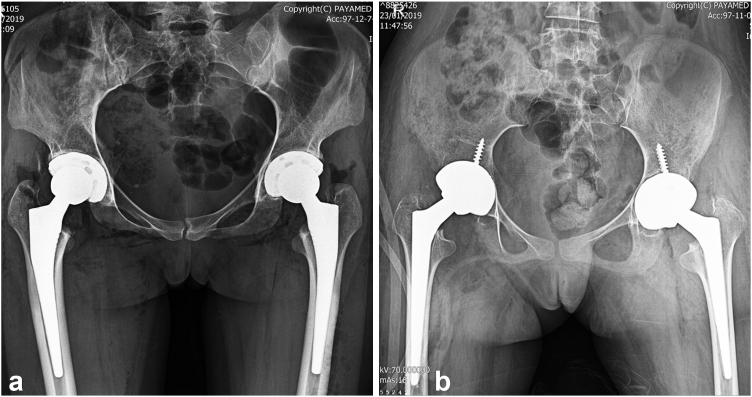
Postoperative anteroposterior pelvic X-rays of patient a (a) and patient c (b) from Figure 1, who underwent THA using a Pinnacle cup and Corail stem (Depuy Synthes) and a Continuum cup and Wagner cone stem (Zimmer Biomet), respectively.
